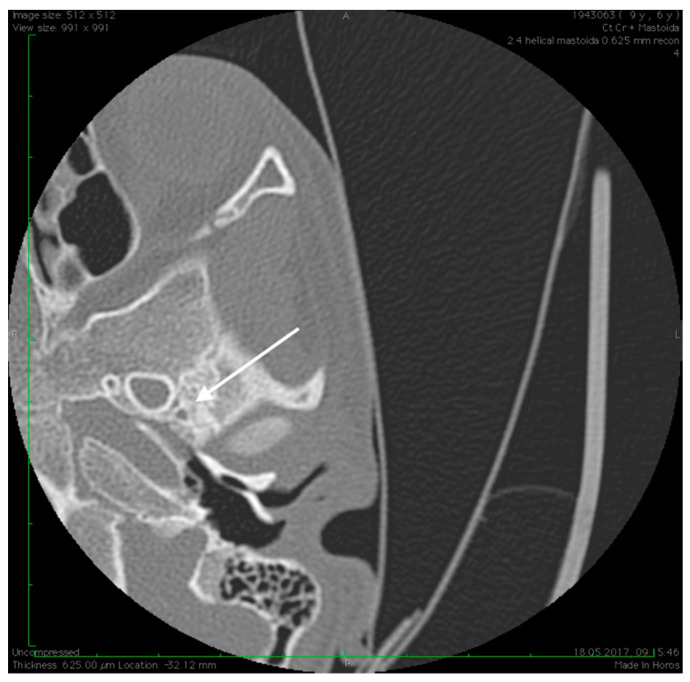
High resolution axial CT scan of left temporal bone exposing the patent foramen spinosum (arrow).
